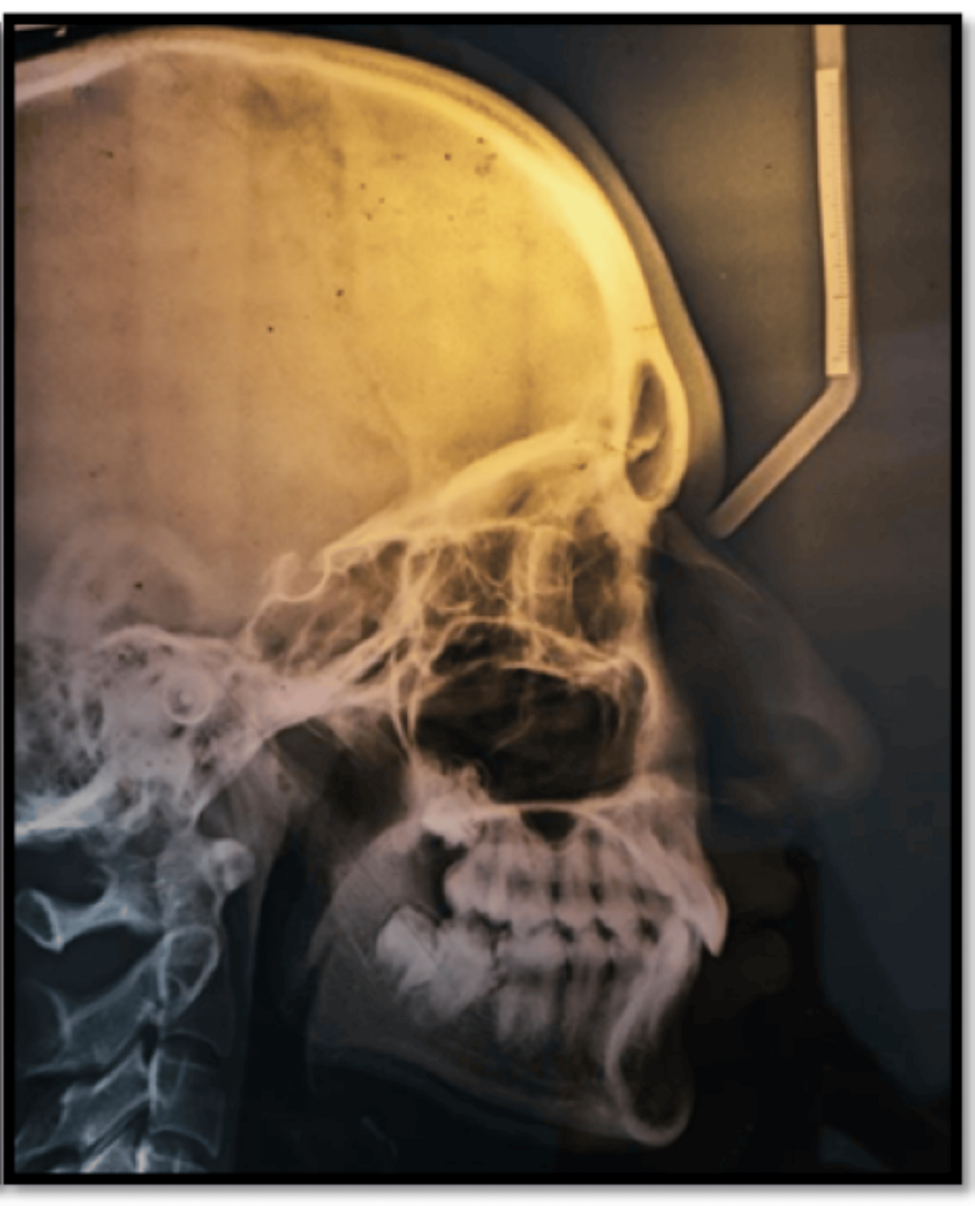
Pretreatment radiograph: lateral cephalogram
